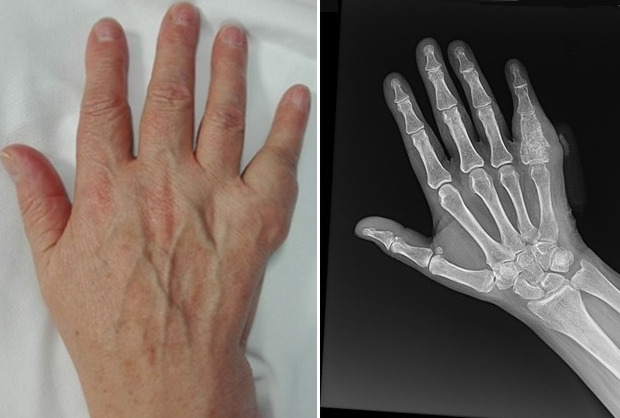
Five years after surgery, the patient returns for pain, swelling and functional limitation; this image highlights the hand and the X-ray with evident recurrence of the pathology
